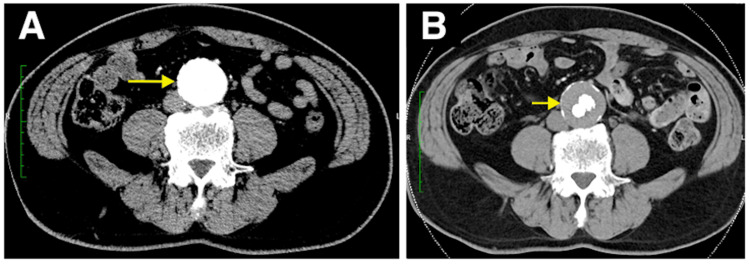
(A) Compared to the computed tomography angiography (CTA) before surgery. (B) CTA of the one-year follow-up after surgery showed sac shrinkage of 10mm.
